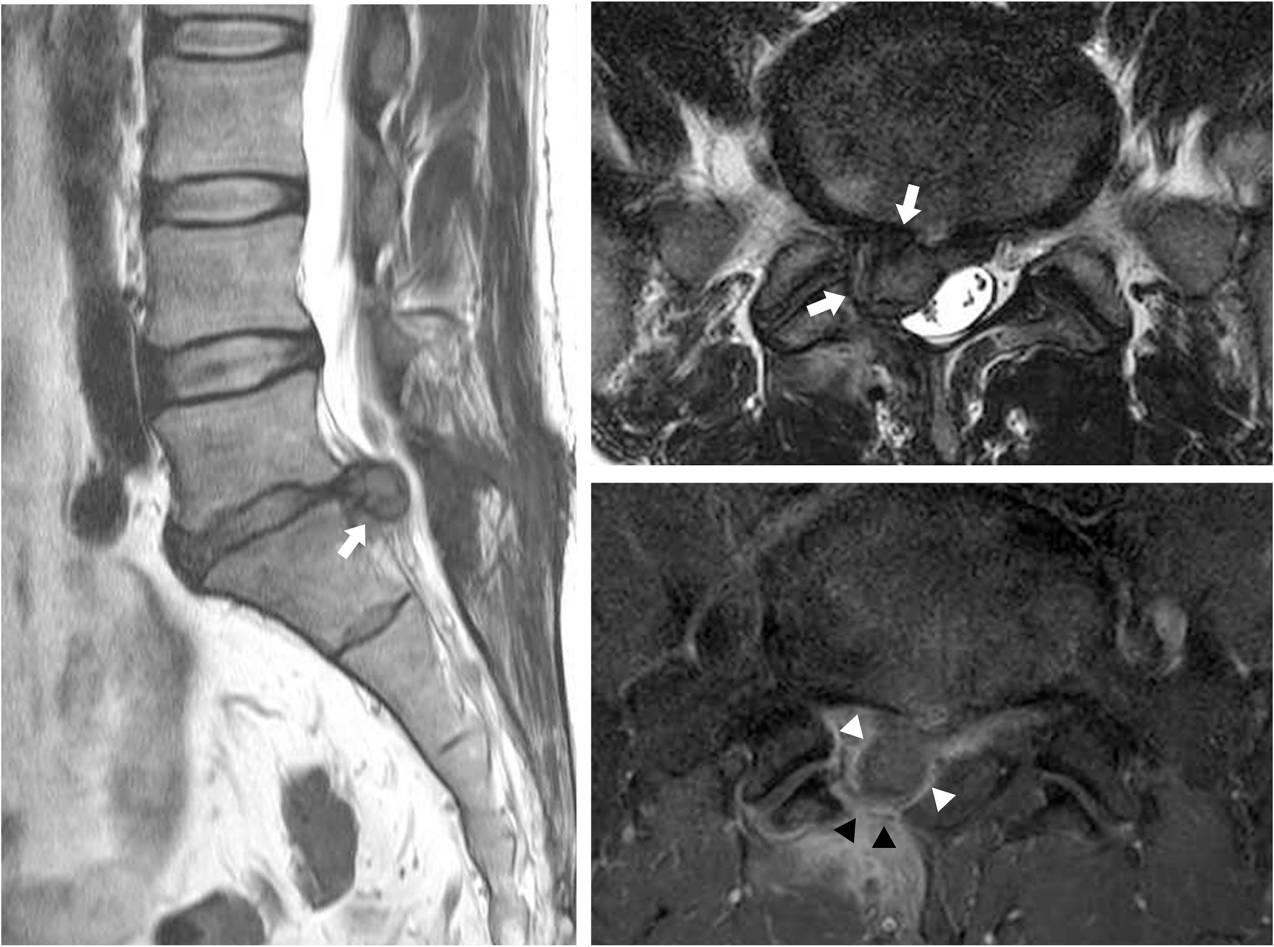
Magnetic resonance imaging of a 41-year-old man with radiating pain to the left buttock and lower leg in the S1 dermatome. T2-weighted sagittal and axial images show recurrent herniated intervertebral disc (white arrows) in the right central zone at the level of L5/S1 with inferior migration causing compression of the right S1 root. The extruded disc (white arrow heads) shows rim-enhancement on the contrast-enhanced T1-weighted axial scan. Diffuse contrast enhancement without mass effect to the spinal canal at the right partial hemilaminectomy site (black arrow heads) of L5 indicating postoperative changes.
